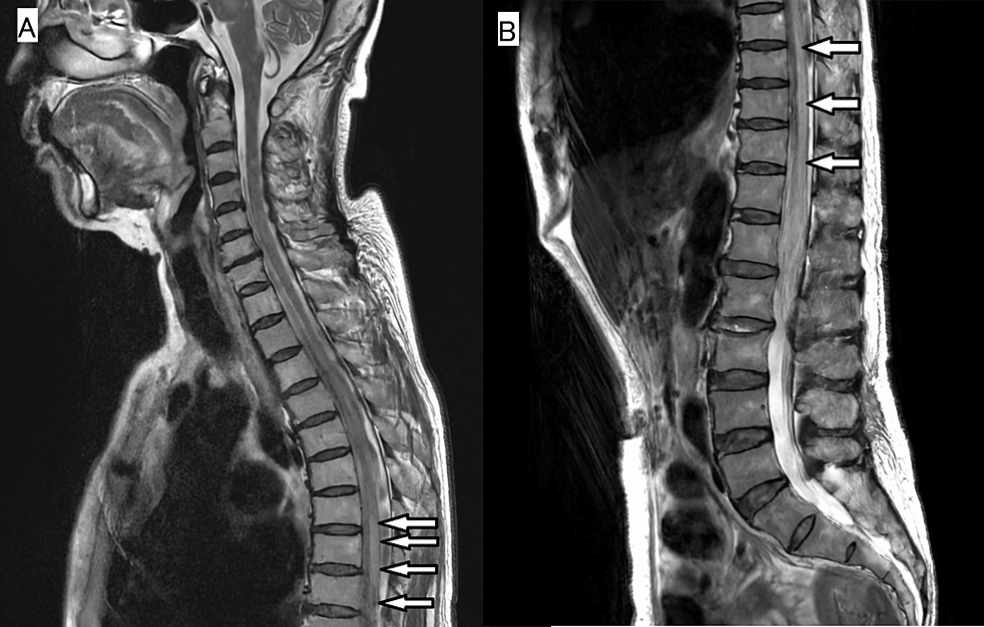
MRI of the spinal cord T2-weighted image shows an intramedullary lesion with high signal intensity from the Th7 level (A) to the spinal cord cone (B)
